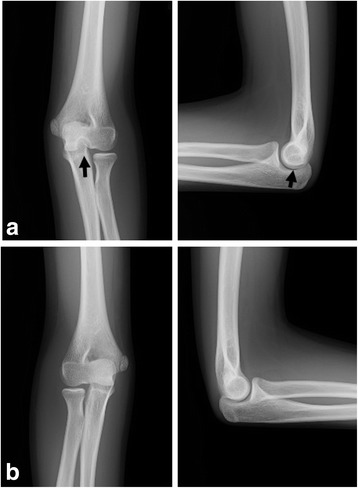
A 14-year-old girl with distal defect of the humerus. Plain radiographs show a well-defined osteolytic lesion with sclerotic rim on the left distal humerus (black arrows). Note that the osteosclerotic rim is absent at the joint periphery (a). The right side of the distal humerus is normal (b)
